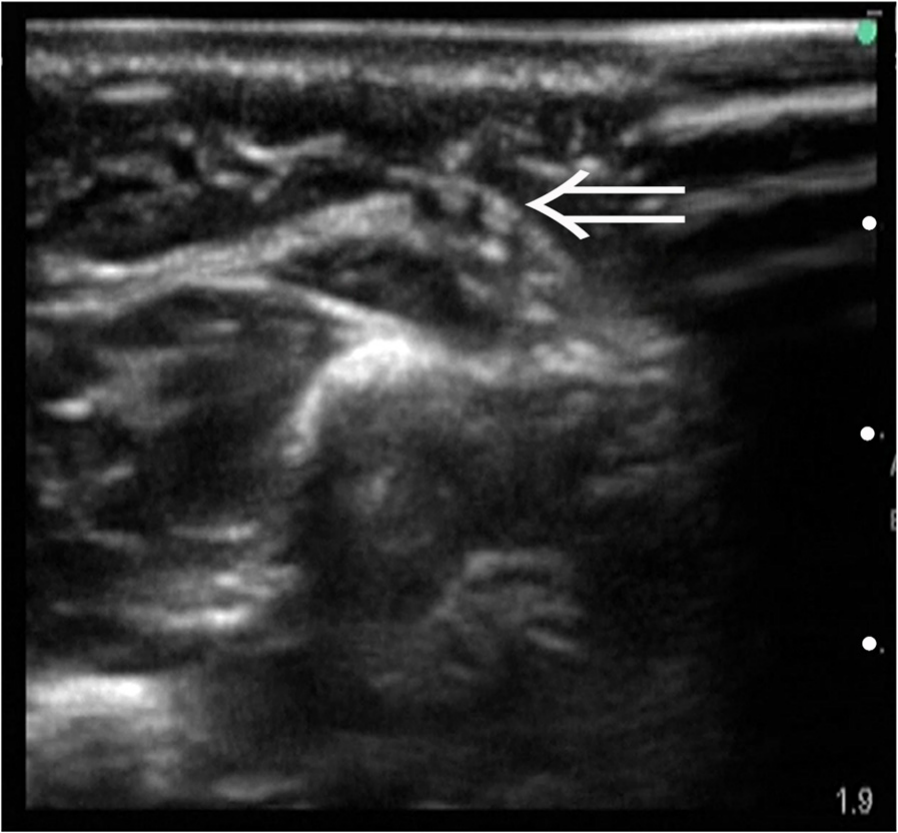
Ultrasound-guided catheterisation for the cephalic vein in the proximal forearm. The 24-gauge catheter tip was visualized in the centre of the vein (arrow). White dots indicate 5-mm increments.
